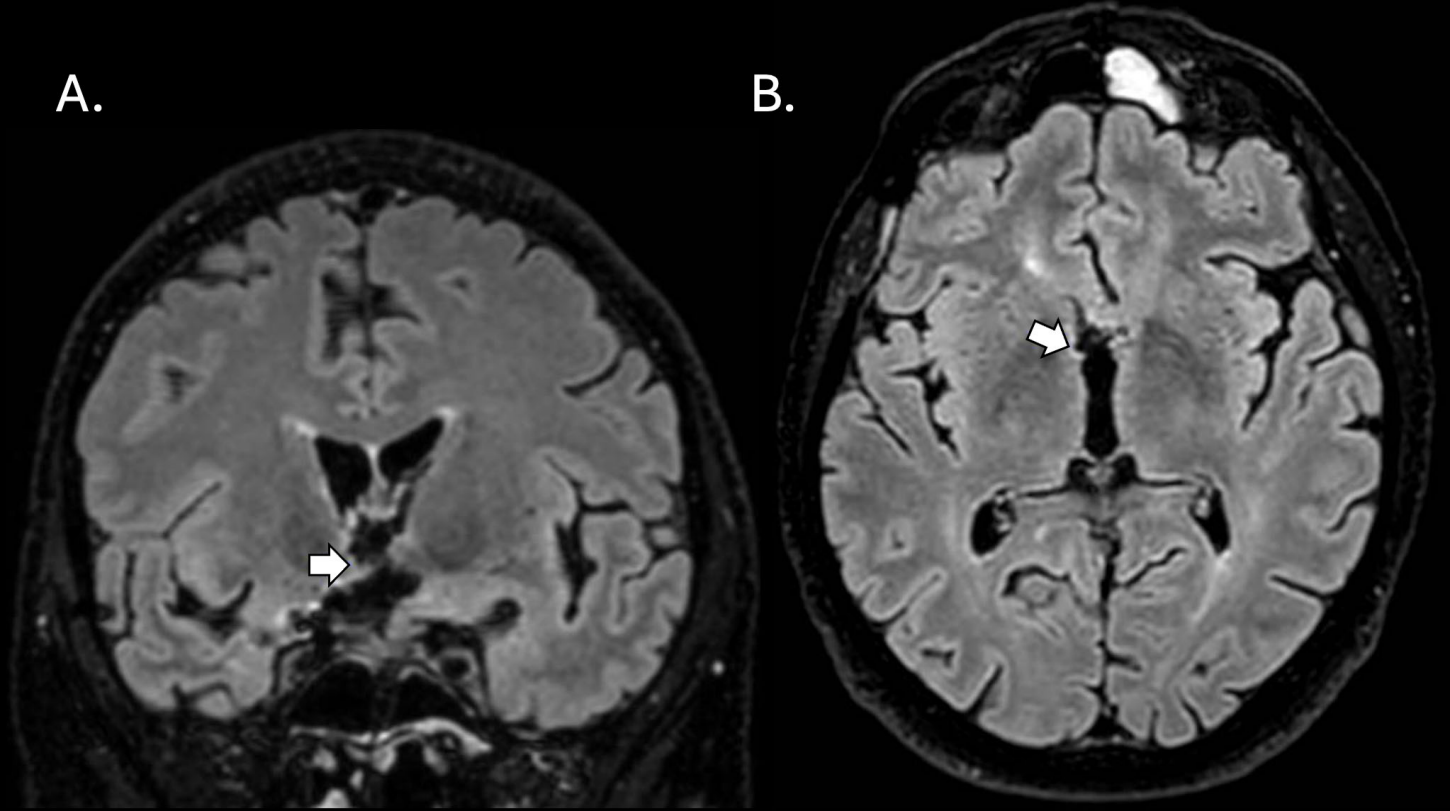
Magnetic resonance imaging (MRI) FLAIR SPIR 1.2mm scans of the brain obtained at follow-up 1 month after the surgical clip ligation. White arrows in coronal (A) and axial (B) planes point to subacute infarction in the right hypothalamus. MRI scans were assessed by a specialist neuroradiologist (J.O.).
